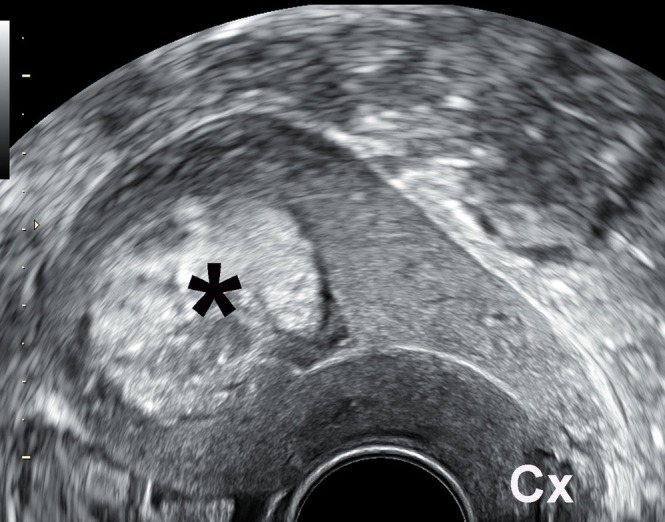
Grayscale transvaginal ultrasound image showing retained placental tissue (
